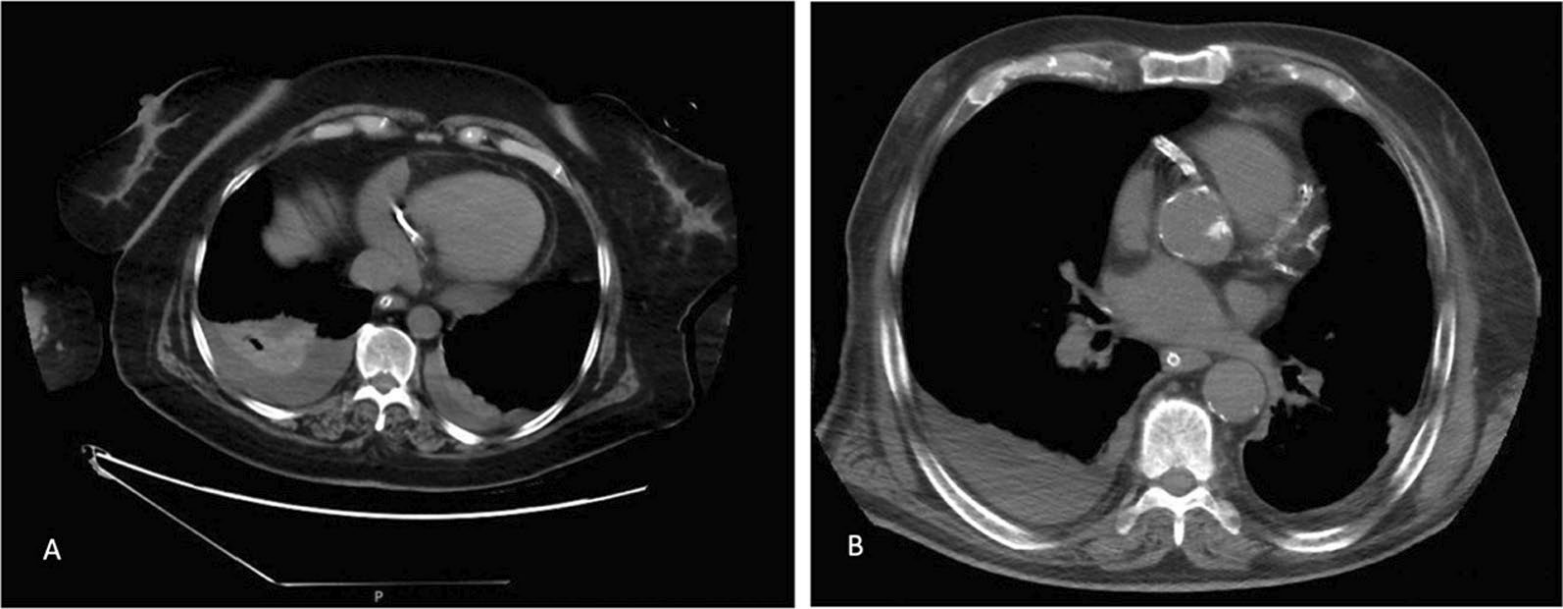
Two different patients showing coronary arteries calcification. A A 59-year-old male with a history of heart attack, non-contrast CT image showing severe, dense coronary calcifications involving the RCA with mild bilateral plural effusion more in the right side with associated atelectatic changes. B A 63-year-old man presented with a history of HTN, smoking and previous stroke, non-contrast CT image showing moderate-to-severe, dense coronary calcifications in the aortic route and involving the left system (LCX and LAD) as well as RCA, mild right-sided plural effusion also noted
